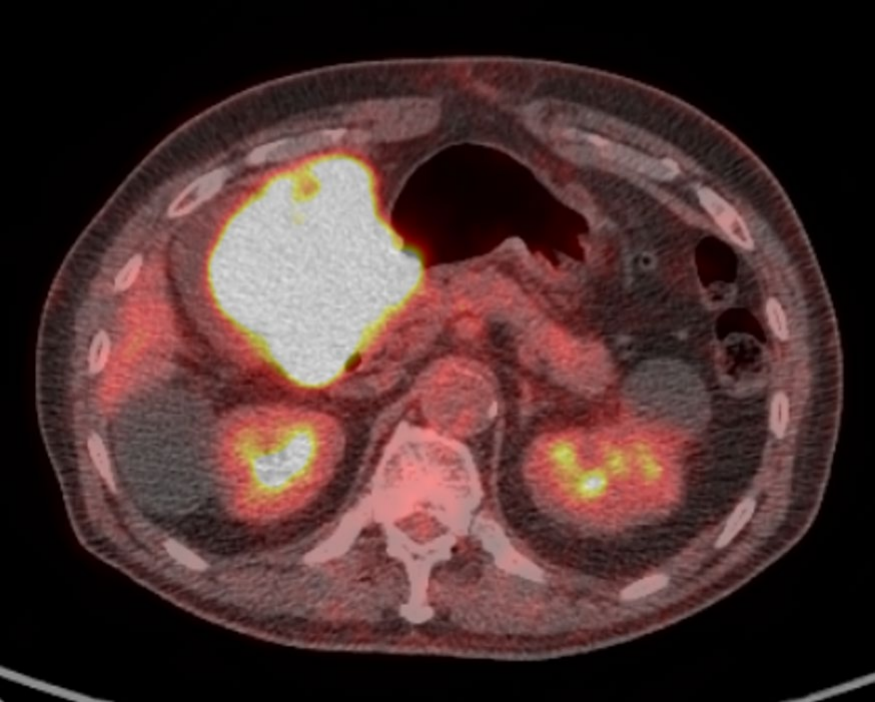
Positron emission tomography/computed tomography scan showed an evidently elevated ^18^F-fluorodeoxyglucose uptake in the lesion site including the tumor thrombus
